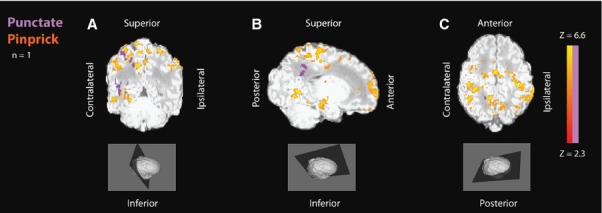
(A–C) Analysis of two accepted 50 mN punctate epochs (purple) and two accepted nontissue damaging pinprick epochs (red–yellow) from the same healthy term infant (infant 19), shown on the infant's own high-resolution structural MRI. Coronal view (A) shows bilateral positive BOLD activation particularly in the primary and secondary somatosensory cortices and the precuneus in response to nontissue damaging pinprick and a smaller areas of contralateral positive BOLD activation in the primary somatosensory cortex in response to vFh punctate stimulation. Sagittal view (B) shows positive BOLD activation in the contralateral side particularly in primary somatosensory cortex and the supplementary motor area, precuneus, occipital and frontal areas in response to nontissue damaging pinprick, and in the supplementary motor area and precuneus in response to vFh punctate stimulation. Axial view (C) shows positive BOLD activation particularly in the parietal cortices, which is much more widespread for nontissue damaging pinprick than for vFh punctate. The coordinates of the three view planes are x:48 (coronal); y:61 (sagittal); z:78 (axial).
